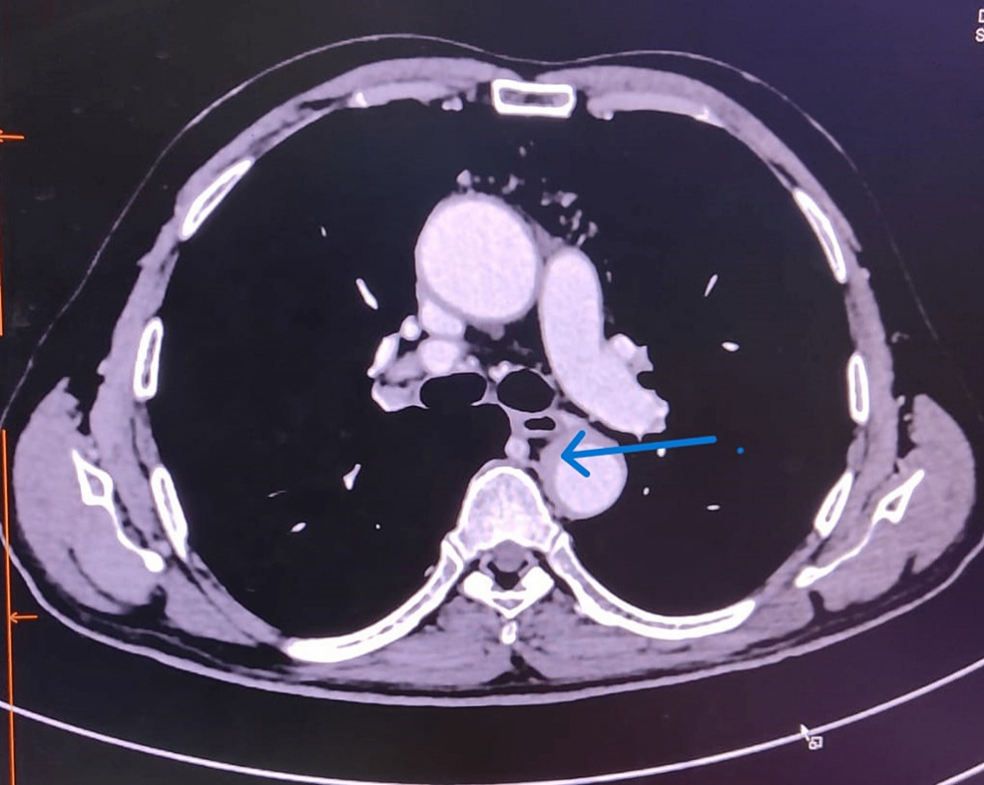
CT angiogram study of the aorta shows an asymmetric circumferential mural thickening (arrow) in the descending thoracic aorta.
